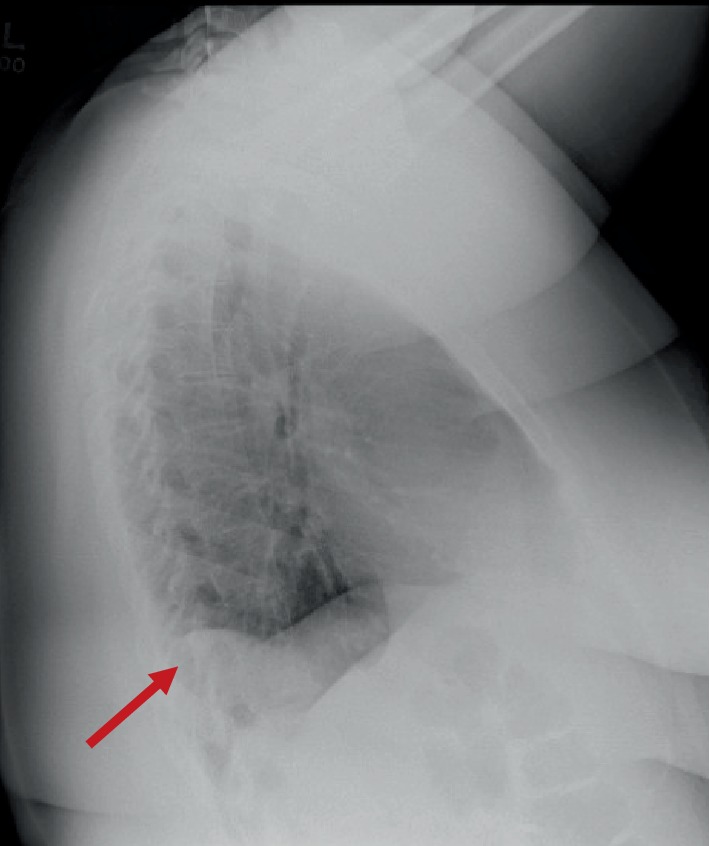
Lateral chest radiograph showing a mass density in the right posterior costophrenic angle with diaphragmatic contour irregularity representing the right side of the bilateral Bochdalek hernia.
